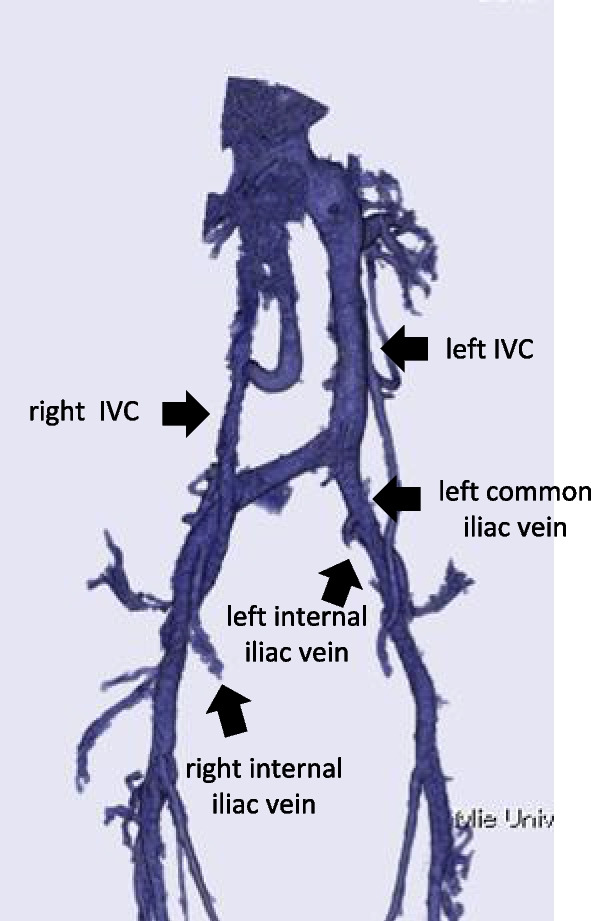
Three-dimensional computed tomography angiography of the inferior vena cava and pelvic veins. No abnormal veins are visualized anterior to the sacrum
